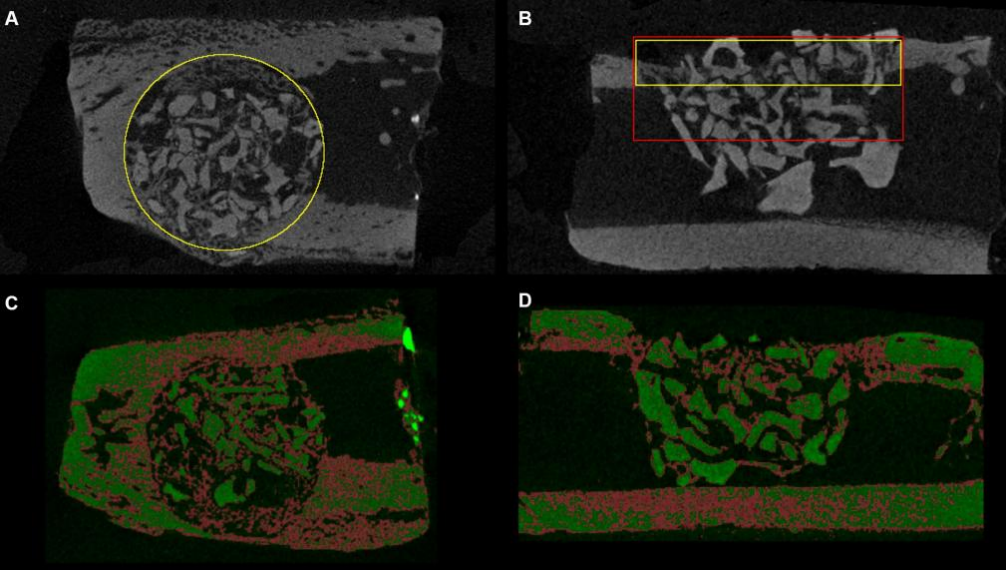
Regions of interest and threshold defined for bone micro-structural parameters analysis by micro-computed tomography. A: Circular ROI defined around the hole defect; B: ROIs including both cortical (yellow) and medullary areas (red); C: Representative threshold applied to transaxial images; D: Representative threshold applied to coronal images.
